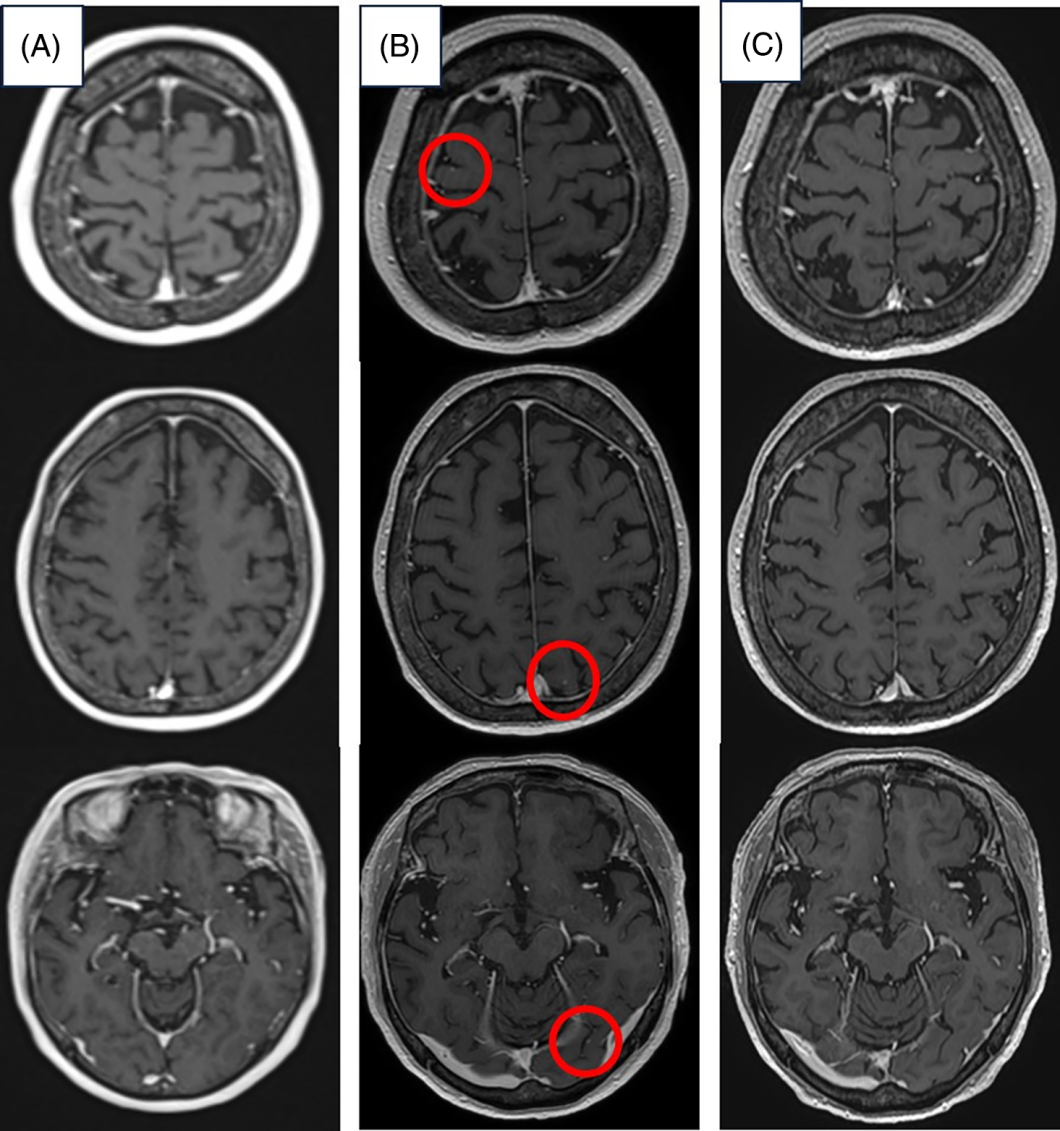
Progress of head MRI. (A) At the time of initial examination. (B) 7 months after the start of treatment. Micro‐nodules can be observed on both sides, which appeared to be brain metastases (red circles). (C) 10 months after the start of treatment, bilateral micronodules have disappeared.
